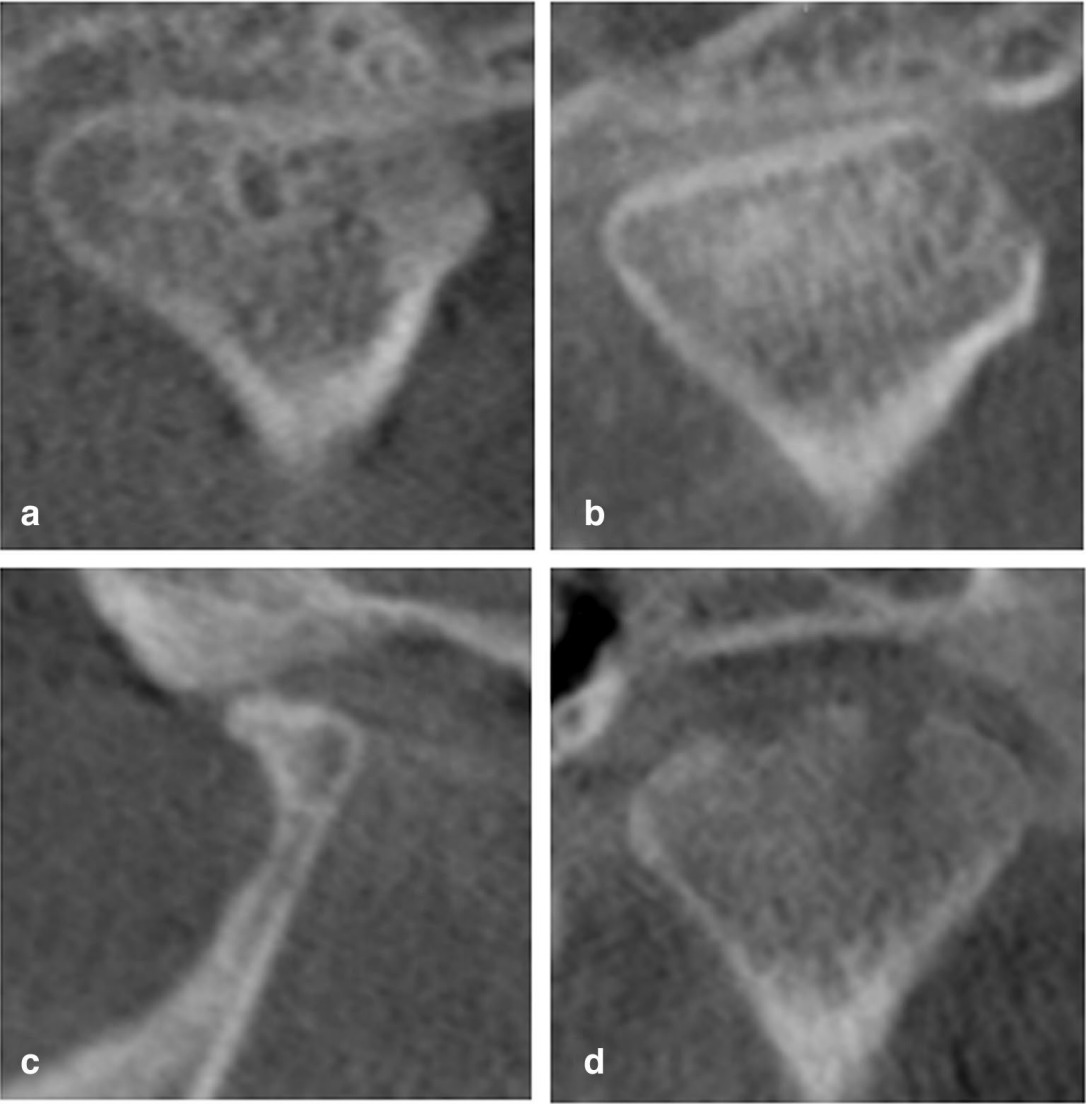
The radiographic criteria used to confirm the diagnosis of osteoarthritis. (a) Subcortical cyst (radiolucent area that may be just below the cortex or in the trabecular bone). (b) Flattening of the articular surfaces (disappearance of the even convexity or concavity of the articular surfaces). (c) Osteophyte (bony outgrowth arising from a mineralized joint surface). (d) Surface erosion (condylar surface irregularities).
